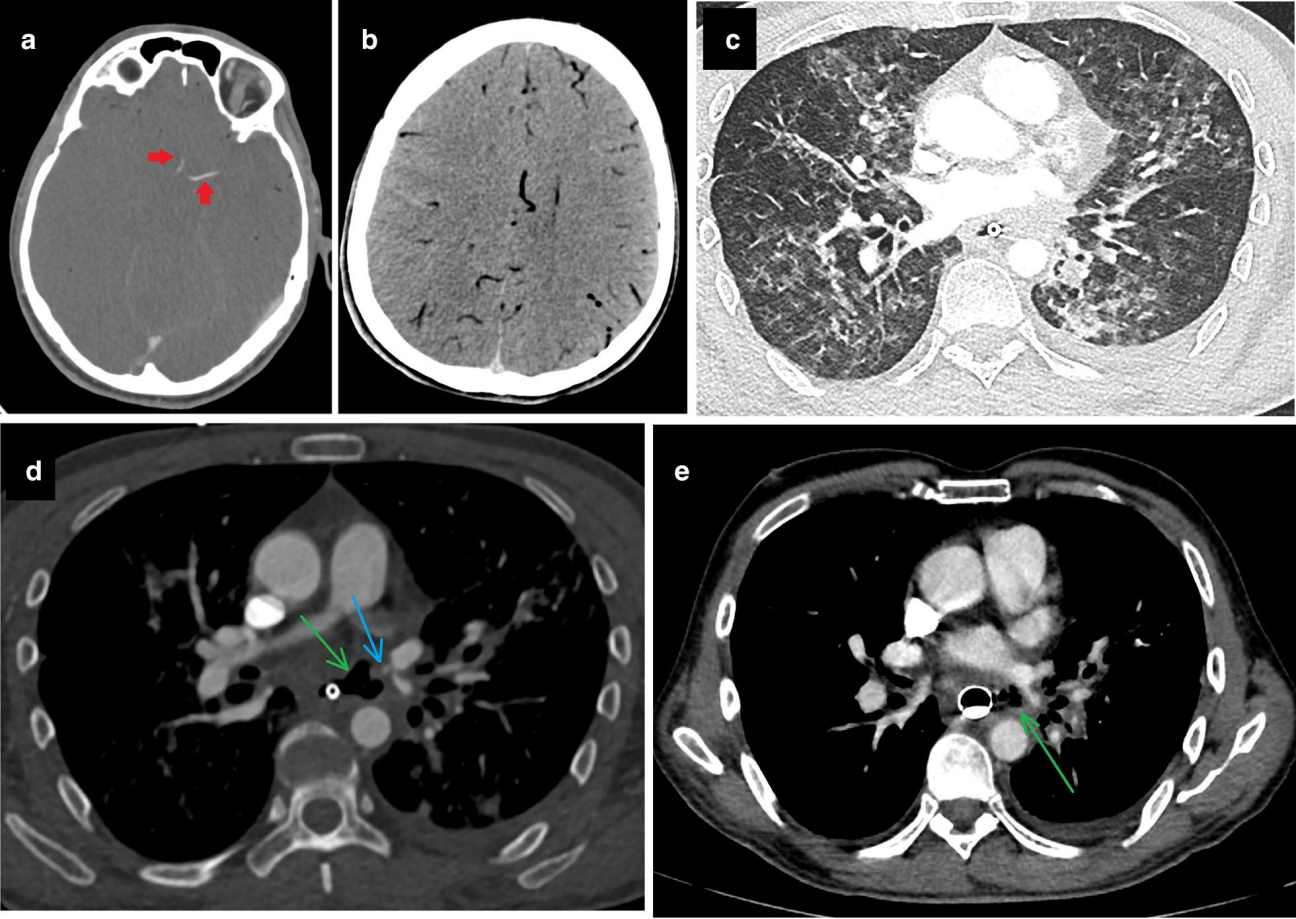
Zebra retreat and hindsight bias. A 75-year-old male patient with known tracheoesophageal fistula (green arrows, d, e) suddenly lost consciousness. Brain CT and CT angiography revealed edematous brain tissue, diffuse air within brain vasculature (b), and contrast filling limited to just proximal segments of the left middle cerebral artery and anterior cerebral artery (red arrows, a). Recent studies of the patient were re-evaluated to detect the etiology of cerebral air embolism. On thorax CT taken 3 days previously for hemoptysis, there was seen to be parenchymal ground glass opacities suggesting hemorrhage (c). In the mediastinal window, next to the fistula, there was an outpouching of the pulmonary vein (blue arrow, d) that had not been present on the previous study (e). The on-call radiologist had also noticed this newly developed outpouching but did not feel confident enough to mention this finding in the report due to the absence of apparent contrast extravasation into the tracheal lumen. Moreover, the radiologist had thought that hemoptysis originating from a pulmonary vein was quite an unexpected situation. This phenomenon is called a “zebra retreat.” It seems to be impossible to overlook or underrate that outpouching with “a priori knowledge of diffuse cerebral air embolism” due to the hindsight bias during retrospective evaluation
